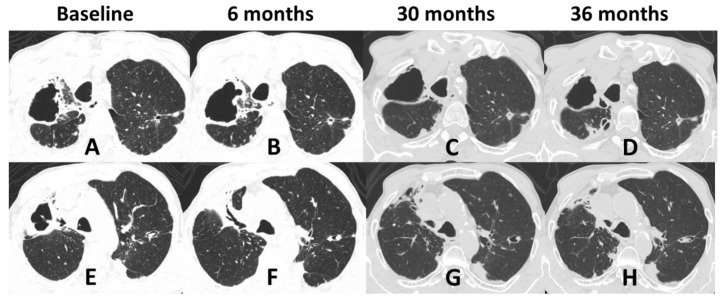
Nonenhanced chest computed tomography of a 63-year-old man with disseminated sporotrichosis and lung lesions, at four moments (Case 2). (A,E) At the beginning of treatment for sporotrichosis. (B,F) Six months of treatment. (C,G) At 30 months of treatment. (D,H) At 36 months of treatment. Among many alterations, there is a thick-walled cavity with irregular margins in the right lower lobe, associated with architectural distortion, fibrotic opacities, and traction bronchiectasis. There is also bronchiectasis in the apicoposterior segment of the left upper lobe, some filled by fluid density material, suggestive of mucous plugging. Areas of pleural thickening in the upper third of the lungs. Images A to D and E to H are similar sections over time.
